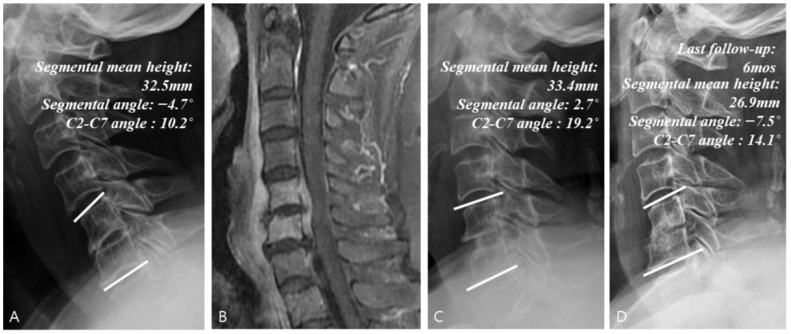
Example of a surgical treatment with no instrumentation case. (A) Simple radiographs of a 68-year-old female patient showing C5–C6 disk space narrowing. (B) Gadolinium-enhanced MR image showing infectious spondylodiscitis at C5–C6, accompanied by prevertebral and epidural abscess. (C) Immediate postoperative plain radiographs showing the state of C5–C6 anterior cervical discectomy and fusion with iliac auto bone graft. (D) Last follow-up (6 months) plain radiographs showing auto bone graft site collapse.
